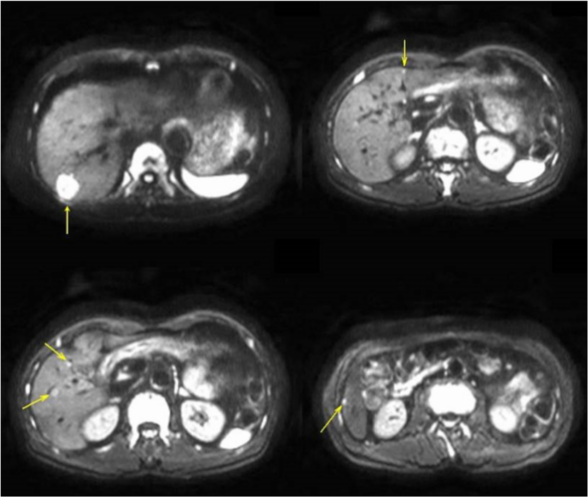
Diffusion weighted MRI of this patient, showing liver metastases in the right lobe and in segment 3.
